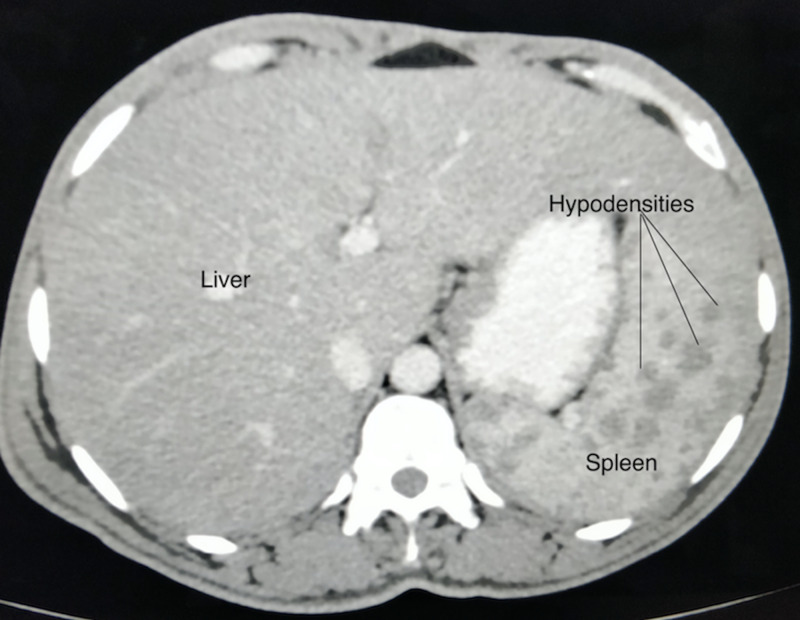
Abdominal CT scan showing splenomegaly with multiple hypoechoic lesions. CT, computed tomography
